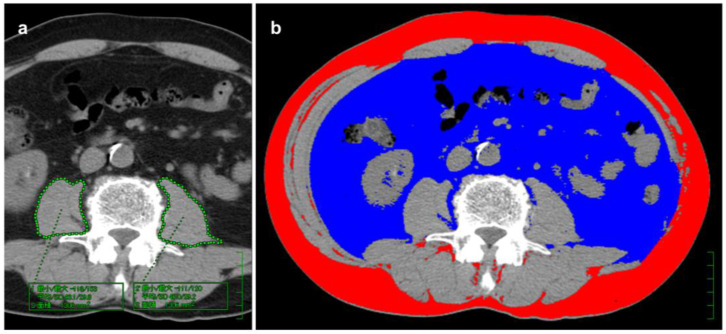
Cross-sectional computed tomography (CT) images at the third lumbar vertebra. (a) Psoas major muscles were semi-automatically traced to calculate total muscle area and mean CT density. Green boxes show measured values (maximum/minimum, mean/SD, and region area). (b) Body fat volume, including subcutaneous (red) and visceral (blue) fat, was measured using Fat Tissue Analyzer 3.0 (Virtual Place Fujin Raijin, Canon Medical Systems, Tochigi, Japan) with thresholds of −190 to −30 HU.
